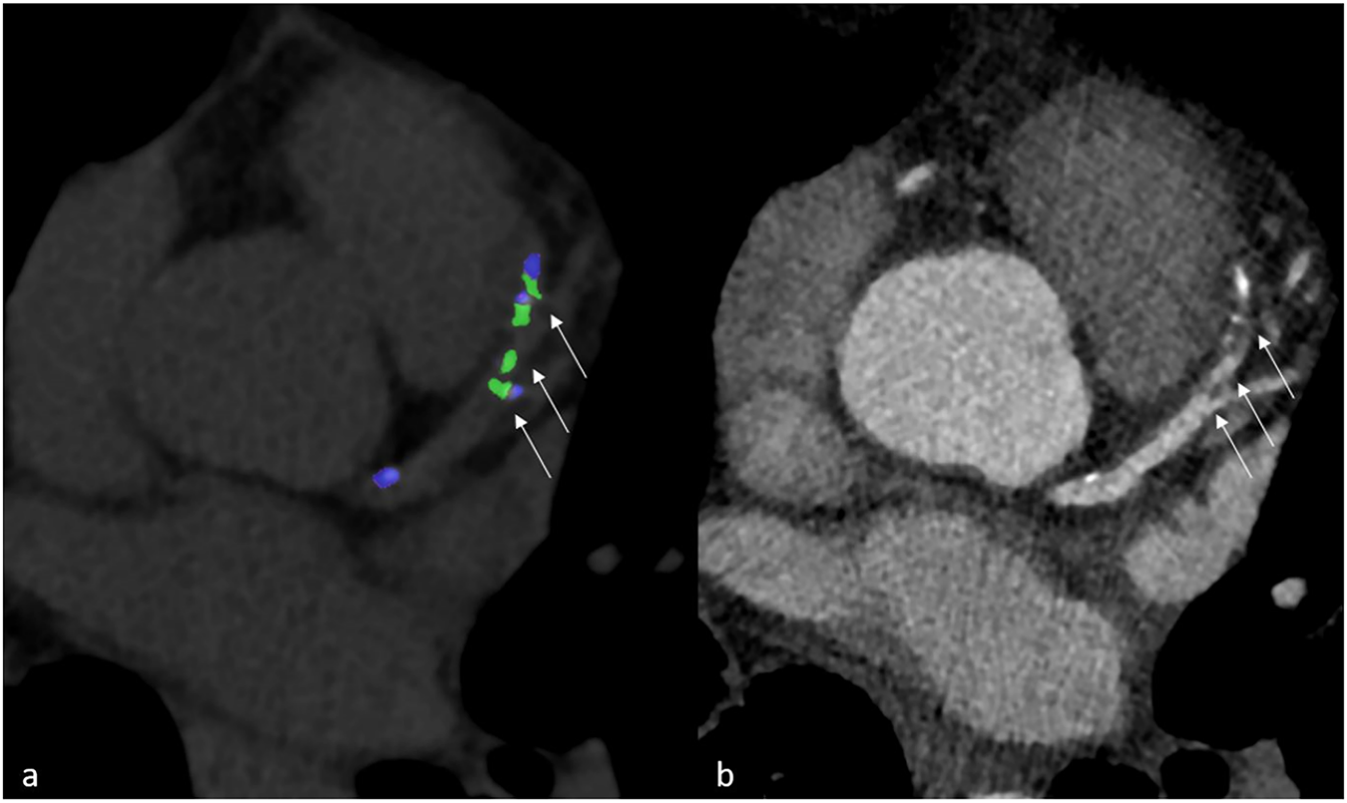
A 75-year-old male with severe tophaceous gout and mixed plaque in the mid LAD coronary artery with HRP criteria. a DECT scan with multiple MSU-positive lesions (arrows). b Coronary CTA showing a high-risk plaque (HRP) with low-attenuation components (mean 57 HU) and positive remodeling (arrows). Blue on DECT (a) calcified coronary plaque
